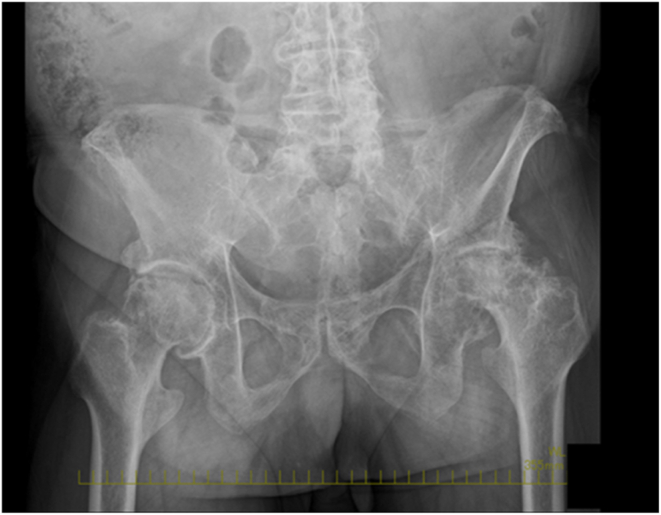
Preoperative pelvic radiograph.
